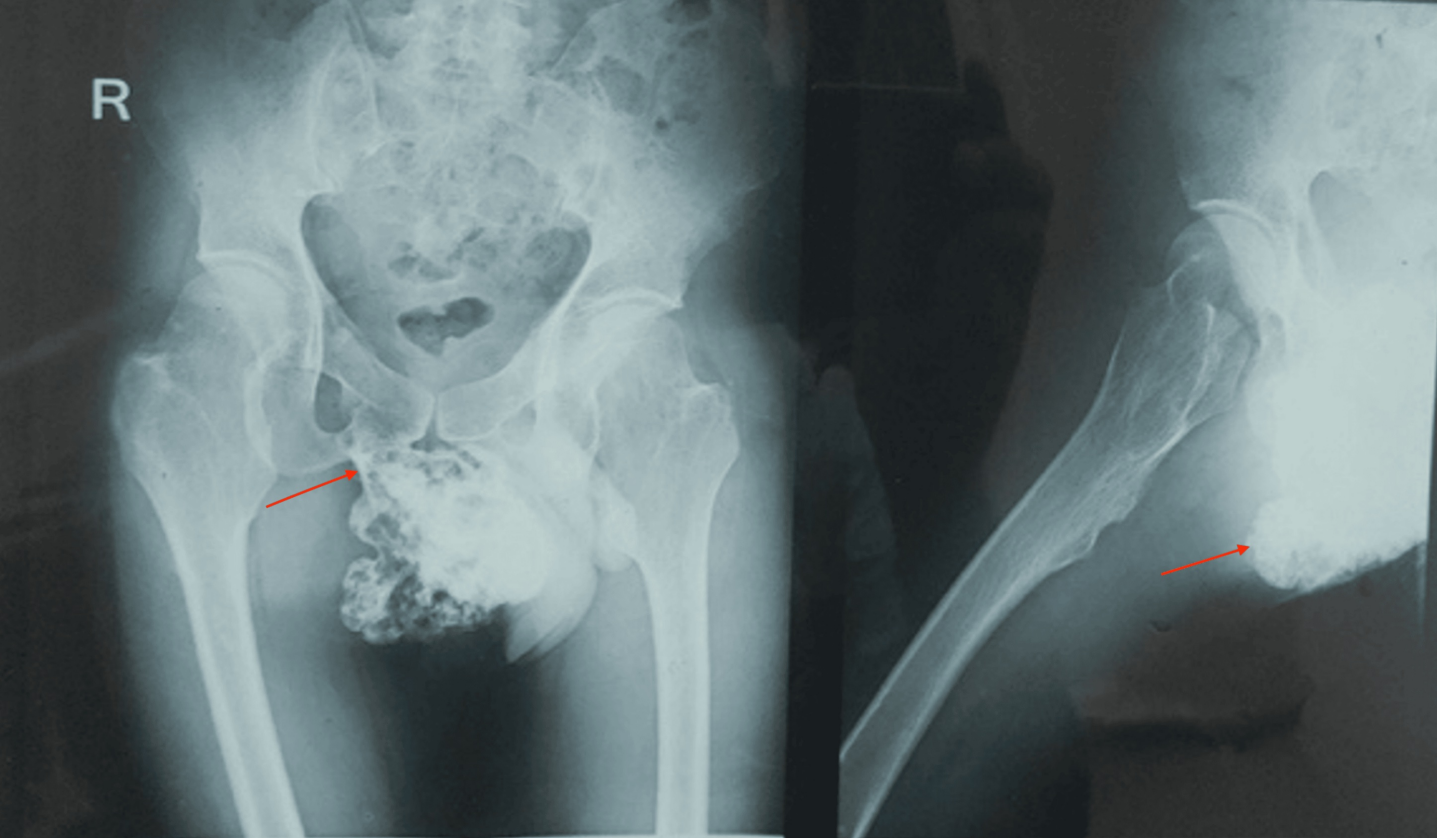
X-ray images of the pelvis Images from anterio-posterior (left) and lateral (right) views showing osteochondroma of the right inferior pubic ramus.
